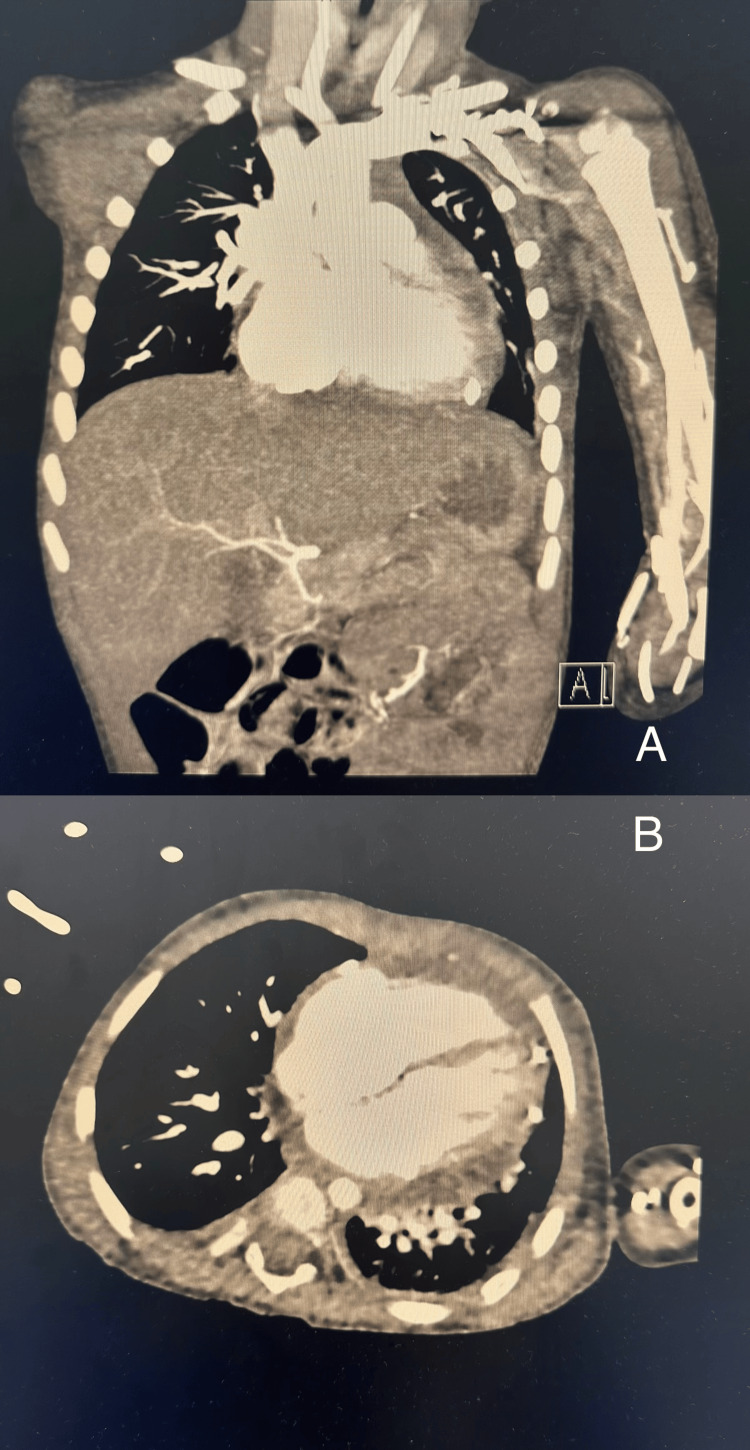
Chest CT Angiography ﻿﻿﻿﻿Panels A and B : Coronal and axial CT views showing large circumferential pericardial effusion without vascular or structural abnormalities. Region of interest (ROI)-based attenuation measurements of pericardial effusion in all views revealed values between 39 and 62 HU, which confirm hemorrhagic fluid. One ROI (179 HU) was excluded as artifact.
